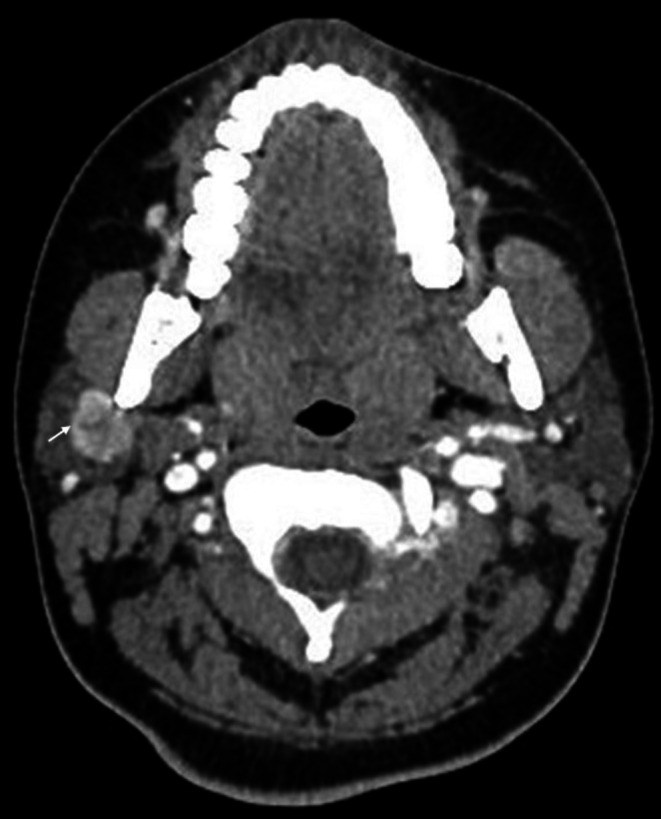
CT imaging revealed a heterogeneously enhancing lesion (arrow) involving the right parotid gland.
